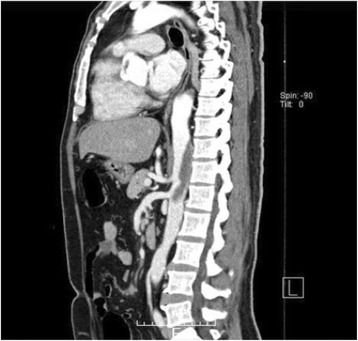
Sagittal view of the preoperative contrast-enhanced computed tomography
